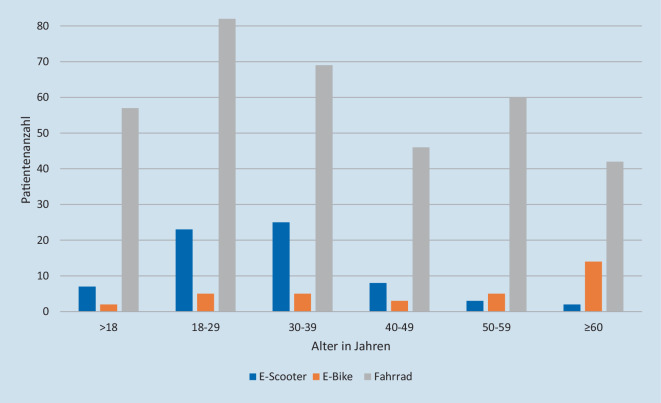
Die am häufigsten betroffene Körperregion bei Verletzungen durch einen E‑Scooter-Unfall war der Kopf/die Halswirbelsäule (Kopf/HWS) mit 38,2 % (*n* = 26), gefolgt von den oberen Extremitäten mit 30,9 % (*n* = 21) und den unteren Extremitäten mit 26,5 % (*n* = 18) (Tab. 3). Die häufigsten Verletzungen der Kopfregion waren Schädelprellungen (*n* = 9), Mittelgesichtsfrakturen (*n* = 8) und intrakranielle Blutungen (*n* = 5). Die genauen Verletzungen der einzelnen Körperregionen sind in Abb. 2 dargestellt. Nur 1,5 % (*n* = 1) der verunglückten E‑Scooter-Fahrer trugen einen Helm. Konventionell radiologische bildgebende Untersuchungen wurden in 51,5 % (*n* = 35) durchgeführt. Eine erweiterte Bildgebung mittels CT wurde in 11,8 % (*n* = 8) der Fälle indiziert. Stationär mussten 70,6 % (*n* = 48) der verletzten Scooter-Fahrer aufgenommen werden, davon 16,6 % (*n* = 8) auf eine Intensivstation, und 29,4 % (*n* = 20) wurden operiert. Die durchschnittliche stationäre Verweildauer (KHVD) nach einem E‑Scooter-Unfall betrug 3 (13) Tage. Der ISS lag bei 13,2 % (*n* = 9) bei ≥ 16. Über den Schockraum wurden 17,7 % (*n* = 12) der Patienten vorgestellt. 66,2 % (*n* = 45) stellten sich selbstständig in der Notaufnahme vor. Von den Patienten mit E‑Scooter-Unfällen hatten 89,7 % (*n* = 61) keine Vorerkrankungen. Unter Einfluss von Alkohol verunglückten 11,8 % (*n* = 8) der Patienten, dies zu 62,5 % (*n* = 5) nachts und zu 100 % (*n* = 8) an Wochenenden oder Feiertagen. 50 % (*n* = 4) gehörten zu der Gruppe der 30- bis –39-Jährigen und hatten ein mittleres Alter von 39,3 (±14) Jahren. Mit einem ISS von ≥ 16 verunglückten 50 % (*n* = 4) der alkoholisierten Scooter-Fahrer, dabei erlitten alle Kopfverletzungen. In Abb. 3 ist die Anzahl der Unfälle mit E‑Scootern im Zeitverlauf von Juni 2019 bis Oktober 2020 dargestellt. Einen ersten Peak konnten wir im Herbst 2019 und einen zweiten im Sommer 2020 verzeichnen. Während des ersten Lockdowns, im Rahmen der SARS-CoV-2-Pandemie, im Frühjahr 2020 gingen die Patientenzahlen nach E‑Scooter-Unfällen stark zurück. Nachmittags und abends ereigneten sich jeweils mit 33,8 % (*n* = 23) die meisten E‑Scooter-Unfälle. Auf dem Hin- oder Rückweg von der Arbeit ereigneten sich 27,9 % (*n* = 19) der Unfälle und wurden BGlich aufgenommen.
